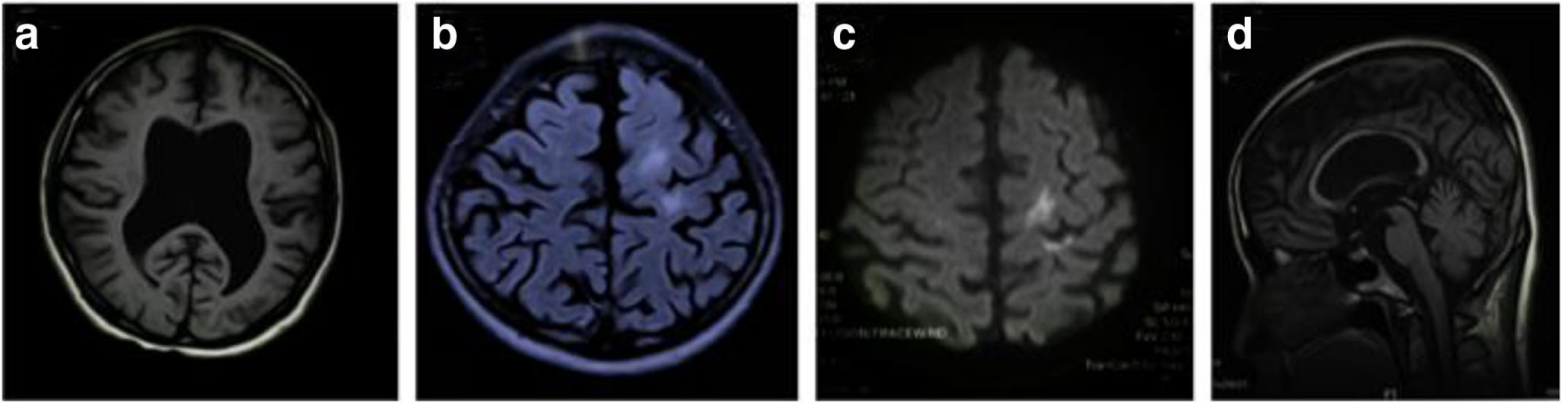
MRI. Neuroimaging of patient III-3. a T1-weighted axial MRI shows mild diffuse brain atrophy and enlargement of the ventricles. b FLAIR axial MRI shows asymmetrical hyperintensity with a frontoparietal cerebral white matter predominance. c Diffusion-weighted imaging (DWI) shows isolated spots of hyperintense signal in the left subcortical frontoparietal cerebral white matter hyperintensity. d T1-weighted sagittal MRI shows brain atrophy and mild thinning of the corpus callosum
